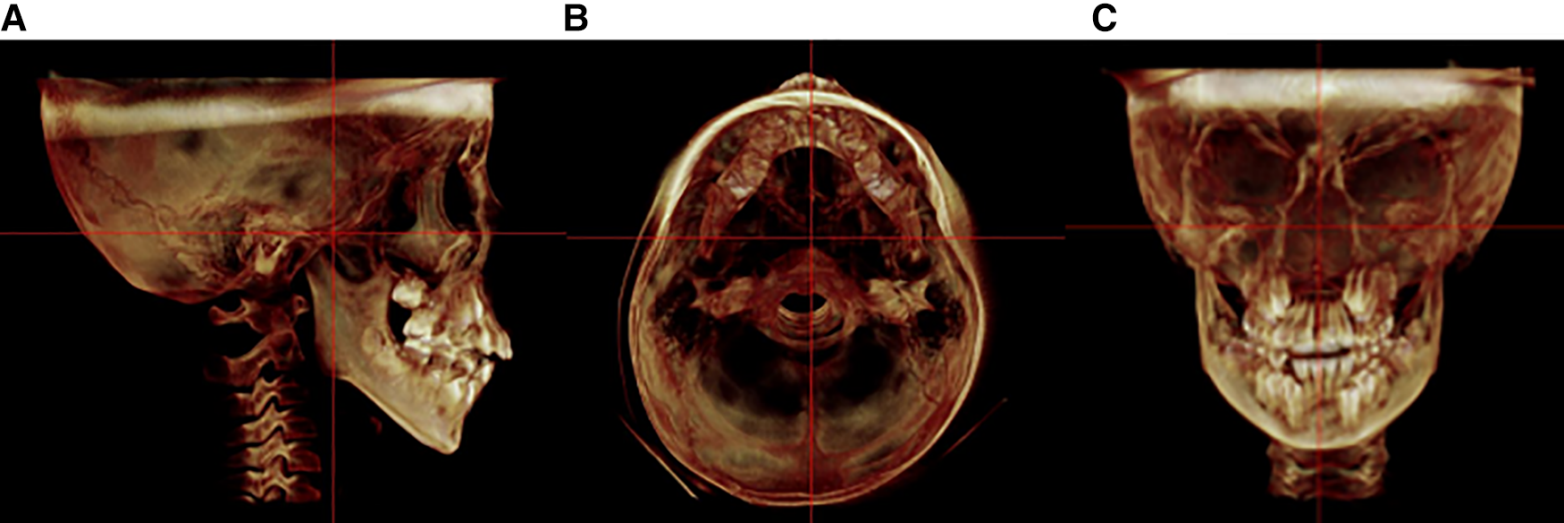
Standardized orientation of CBCT for measurement. (A) Sagittal plane. (B) Horizontal plane. (C) Coronal plane.
